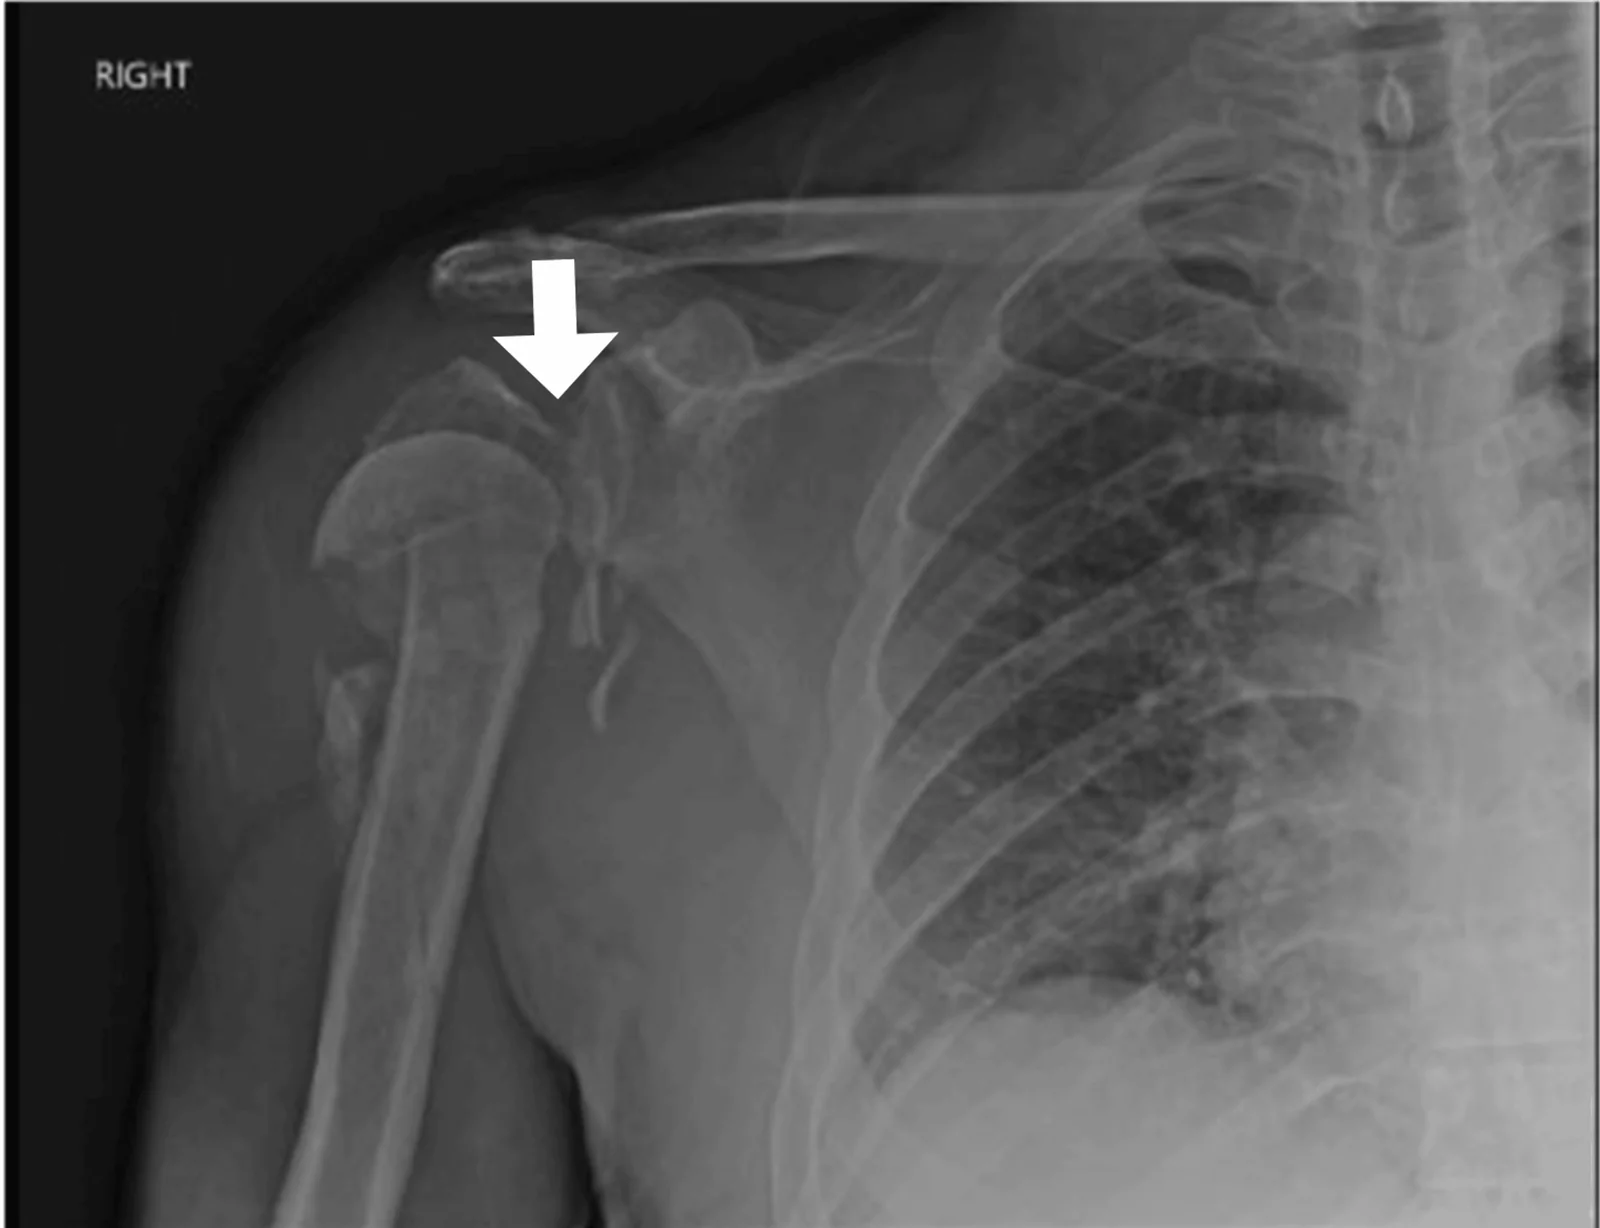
Right shoulder radiograph in internal rotation demonstrating severe comminution and malunion/nonunion of the proximal humerus White arrow points to the comminution of proximal humerus.
